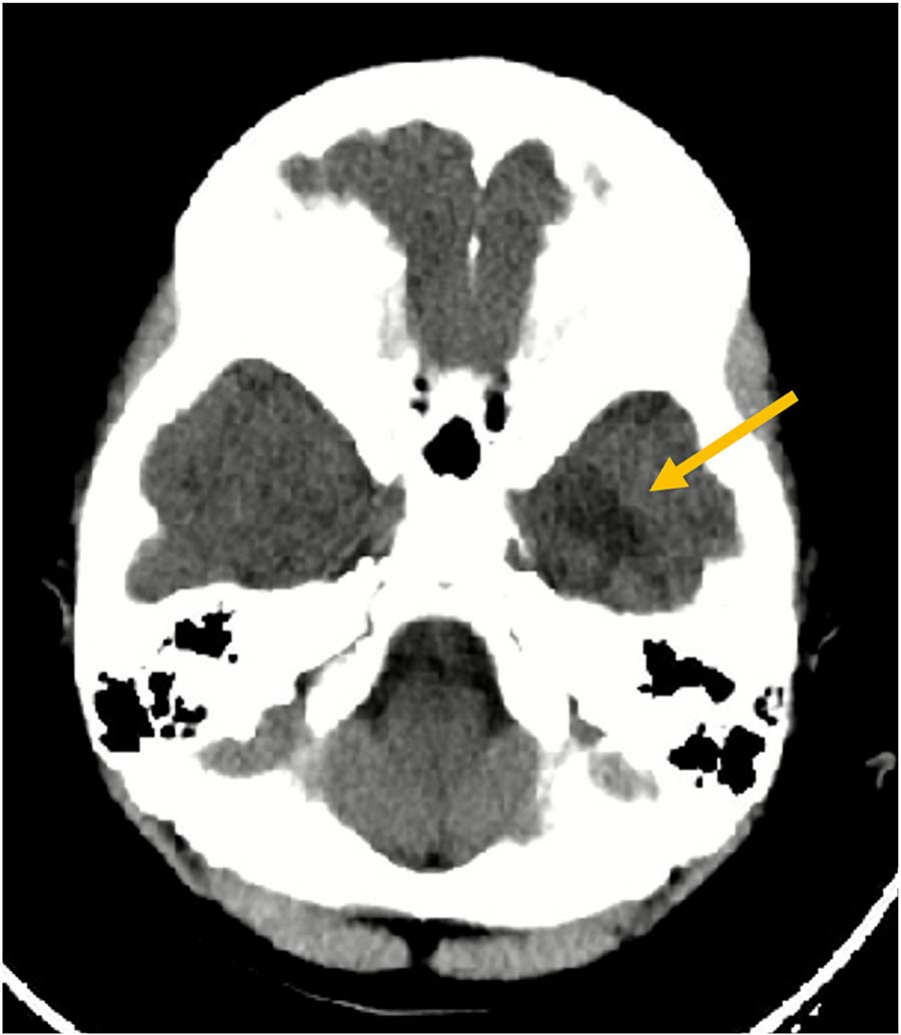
Axial non-contrast CT scan of the brain. This image demonstrates an ill-defined hypodensity (arrow) in the left inferior temporal lobe (seen as a darker area). No acute intracranial hemorrhage or fracture was identified at the time of this initial scan.
